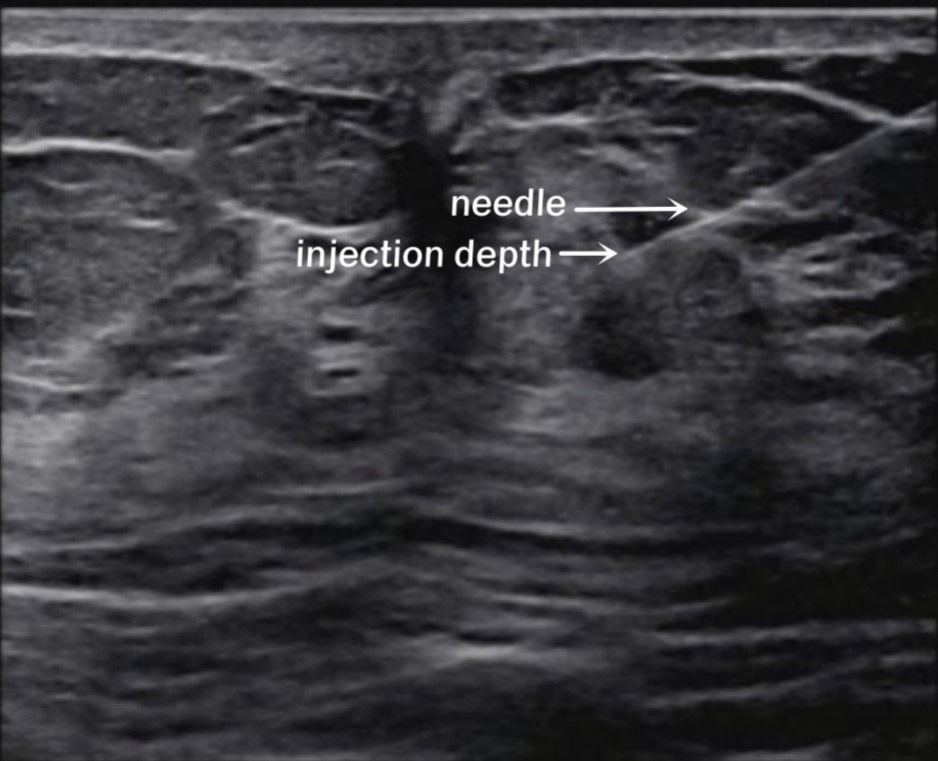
Ultrasound-guided intraglandular injection around the areola. The white arrows show the needle and the injection depth (6 o’clock).
